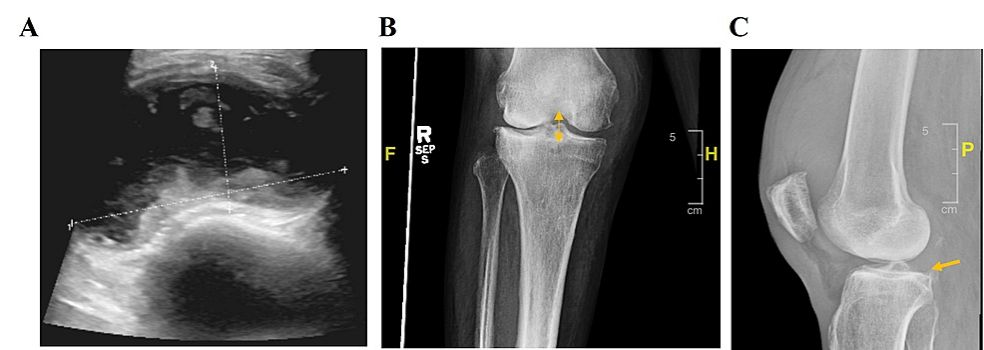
Representative images of the affected knee (A) Ultrasound of the right knee showing a complex large effusion measuring 12 × 7 × 3 cm (white lines). (B and C) Two view radiographs redemonstrating large effusion. Narrowing of the lateral tibiofemoral joint space (B: yellow double-headed arrow) and minimal articular surface bony spurring is noted in the patellofemoral and tibiofemoral joints (C: yellow arrow).
